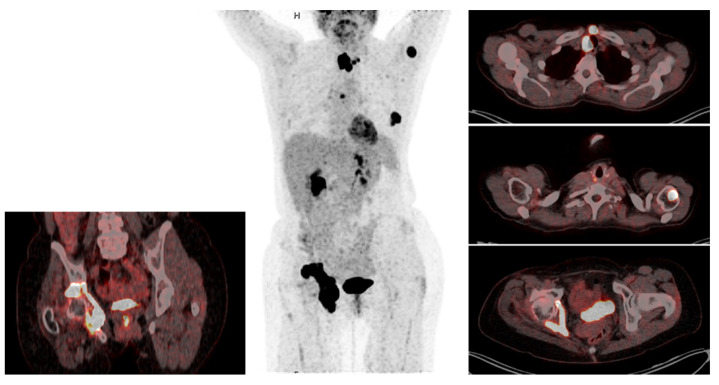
Cervical surgery in the setting of metastatic disease. This 55-year-old female patient underwent R0 resection of a minimally invasive pT3 cN0 cM0 FTC with vascular invasion 2014 and received her first RAIT. She first presented at our university clinic two years later, when she was diagnosed with cervical recurrence. She underwent cervical surgery, followed by cervical EBRT for diffuse soft tissue micrometastatic disease. Three years later, she was diagnosed with a new cervical relapse despite EBRT and a pelvic metastasis, which was deemed not resectable by orthopedic surgery. After her third cervical surgery in 2019, revealing soft tissue metastatic disease, she received a second RAIT and pelvic EBRT. These are the 18F-FDG PET scan and die DWBS six years after begin of disease. In addition to the pelvic metastasis and a further cervical relapse, new bone metastases are visible. The patient underwent her fourth cervical surgery this year. The trachea resulted superficially infiltrated over several cm, making a tracheal resection in the setting of metastatic disease not reasonable. A shaving resection was performed. Lenvatinib, which had been started 2020, was not tolerated well by the patient and treatment was discontinued. Disease is currently slowly progressing.
